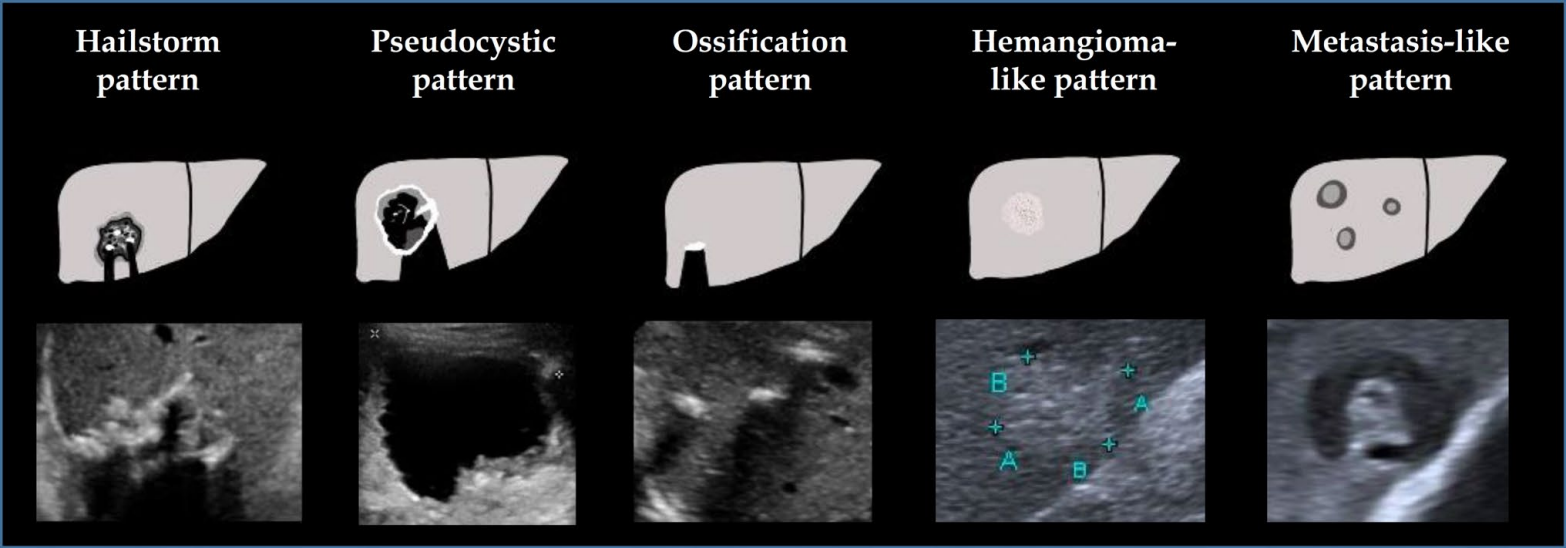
Echinococcus multilocularis* Ulm classification—ultrasound (EMUC-US) [10]
